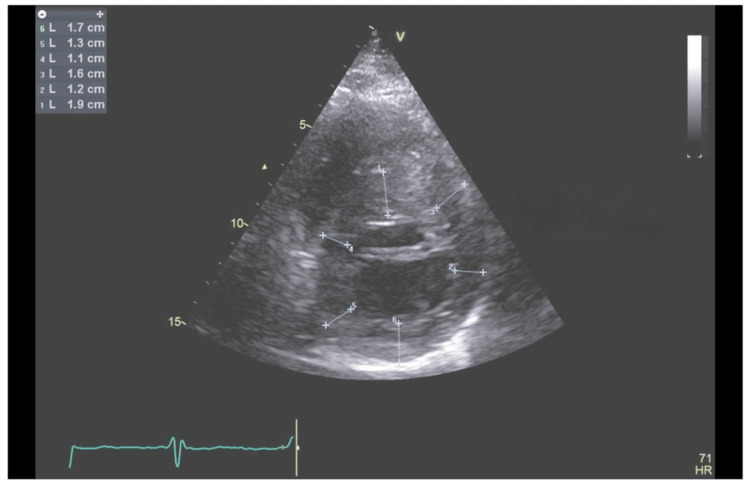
Echocardiogram - parasternal short-axis view with wall thickness measurements Multiple measurements showing septal thickness ranging from 1.1 to 1.9 centimetres confirming septal hypertrophy.
